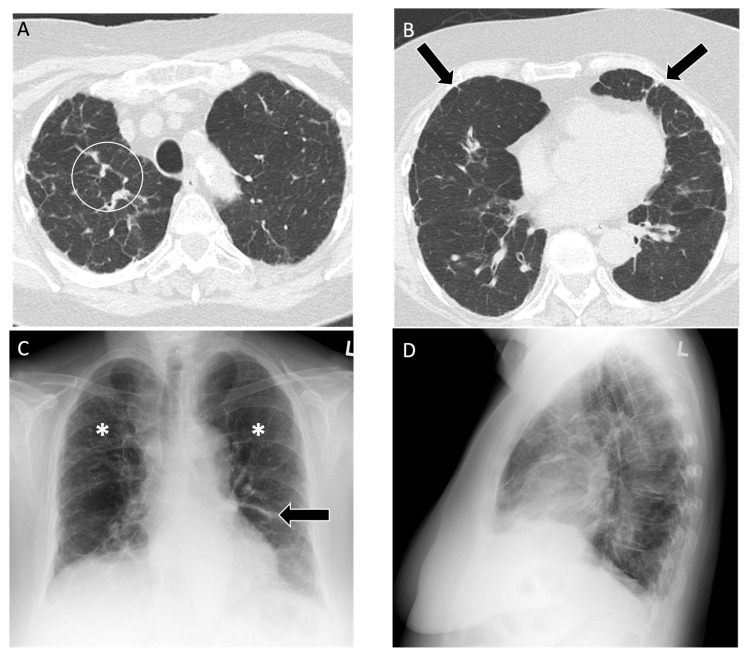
A 76-year-old non-smoking female was diagnosed with farmer’s lung, proven with biopsy. Computed tomography of the upper lobe demonstrates (A) peribronchovascular reticulations (white circle) with mosaic lung attenuation and (B) mild diffuse subpleural reticulations (black arrows); (C) posteroanterior and (D) lateral view radiographs demonstrate diffusely coarse reticulations and fibrosis (asterisks) in the upper lung zone with some mild volume loss. A staple line in left lower lobe is noted from a previous lung biopsy for diagnosis of farmer’s lung disease (black arrow).
